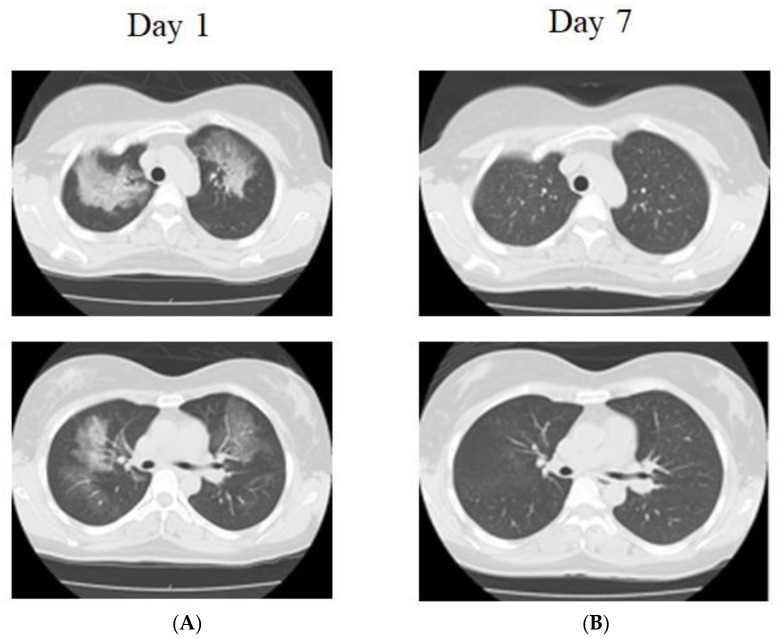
Chest X-ray and chest computed tomography (CT) in case 2. (A) Bilateral diffuse, ground-glass opacity predominates in the upper lung fields, with a partially mosaic pattern, and no pleural nadir shadow. There is no pleural effusion (Day 1). (B) Improved completely (Day 7).
